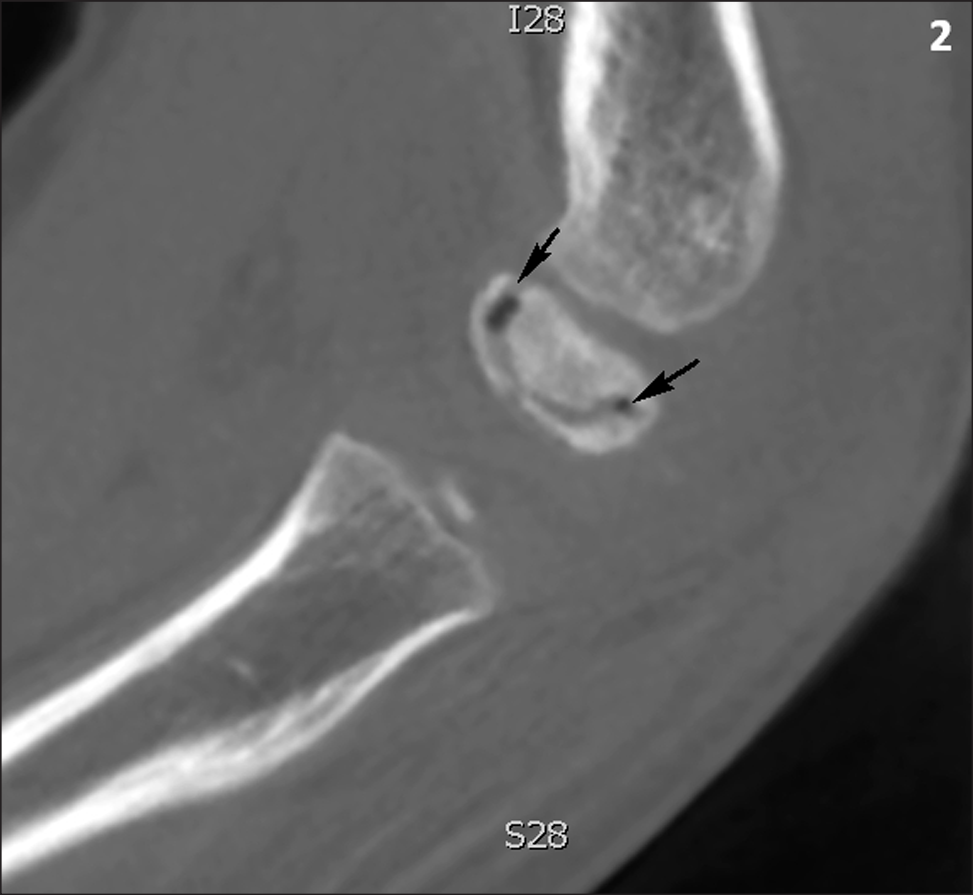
Cone beam CT of the right elbow, sagittal reformatted image. The capitellum has an increased density. There is a subchondral crescent-shaped vacuum phenomenon (black arrows).
